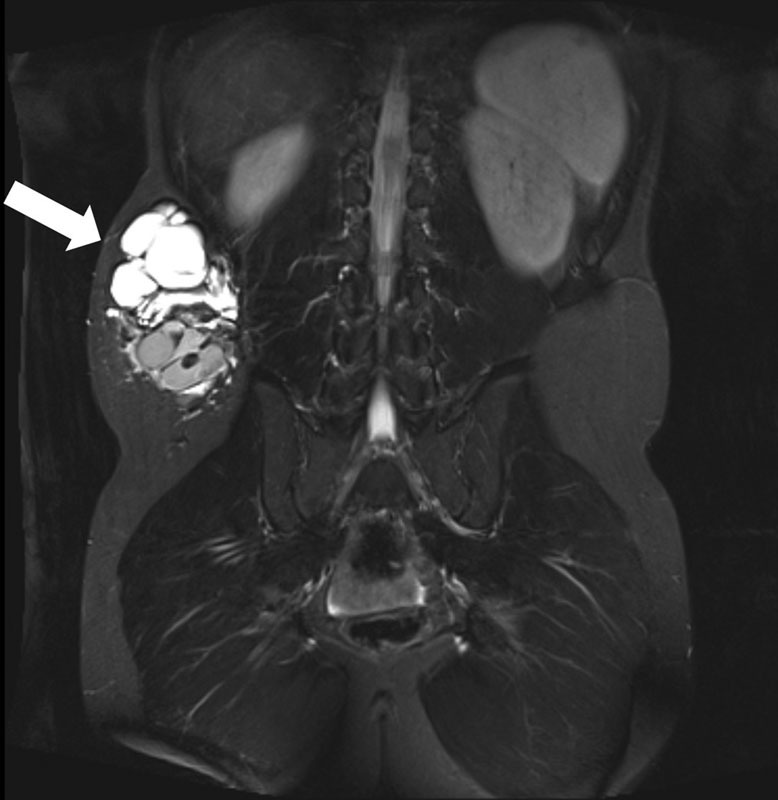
Coronal T2 magnetic resonance imaging (MRI) with a subcutaneous mixed macro-/microcystic lymphatic malformation on the patient's right flank (white arrow).
